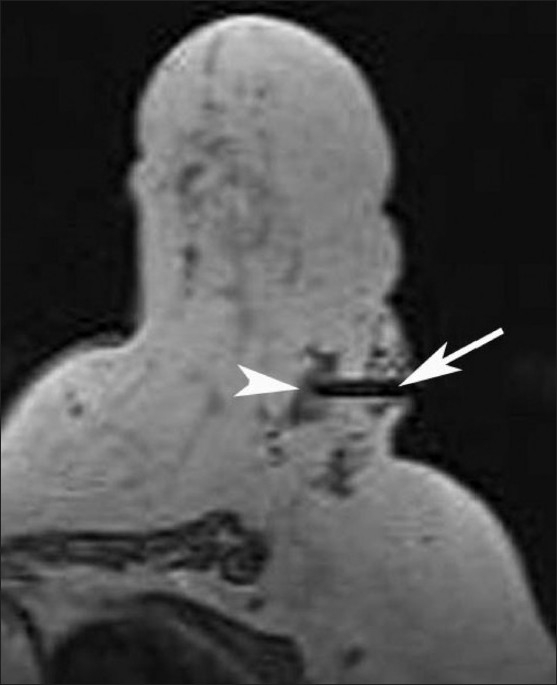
MRI-guided localization. Axial, T1W non– fat-suppressed MRI image shows a thin magnetic susceptibility artifact (arrow) due to the localization wire in place. The lesion (arrowhead) is seen near the tip
